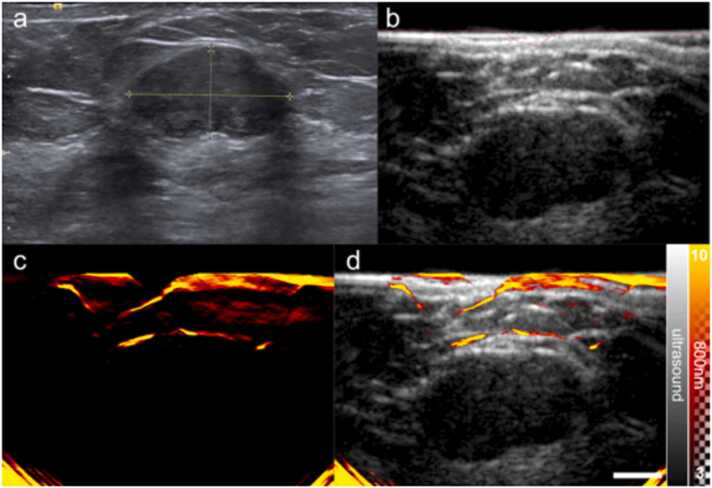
A BI-RADS 3 lesion successfully downgraded by OA-US. (a) Standard-of-care ultrasound reveals an oval hypoechoic mass, with few lobulations seen, hence on B mode US alone the lesion was classified as BI-RADS 3. (b) US image from the OA-US scan at the same position reveals an oval hypoechoic mass (c) The OA image from the OA-US scan at 800 nm reveals blood vessels, which appear draped over the lesion in the overlaid OA-US image (d) and would downgrade the image to BI-RADS 2. Scale bar 1 cm.
